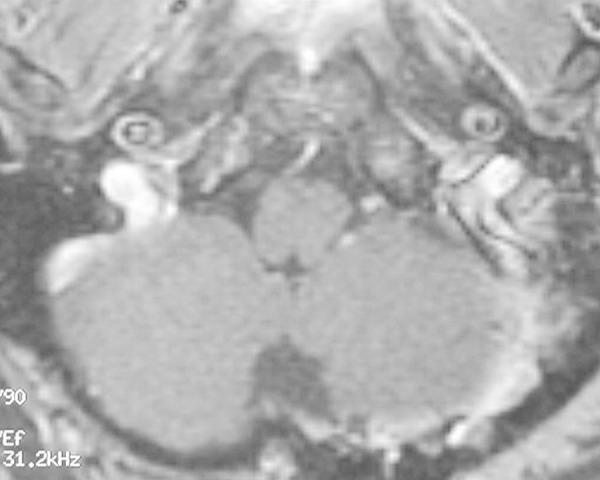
Postoperative MRI of Case 1 Postoperative MRI of Case 1 depicting an apparent total gross resection of the lesion.
